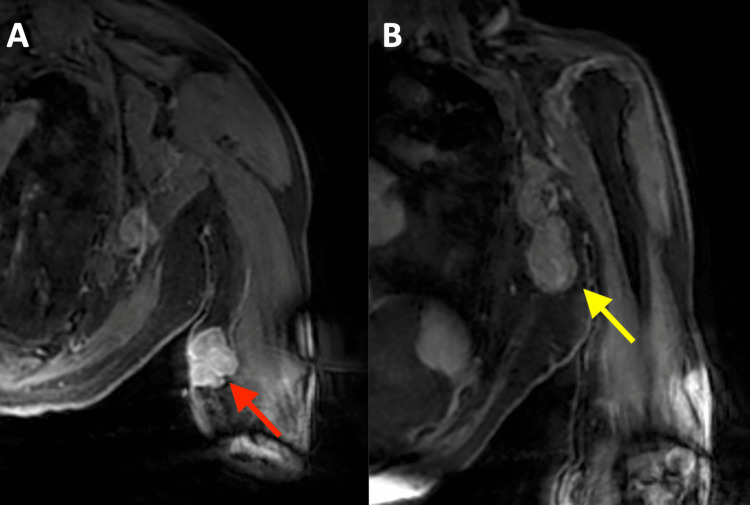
A. Coronal image of a post-contrast T1-weighted MRI sequence with fat suppression of the left upper extremity revealing an avidly enhancing polypoid mass (red arrow) within the soft tissues overlying the left triceps musculature. B. Additional coronal image of a post-contrast T1-weighted MRI sequence with fat suppression demonstrating multiple enlarged and enhancing left axillary lymph nodes (yellow arrow). MRI: magnetic resonance imaging
